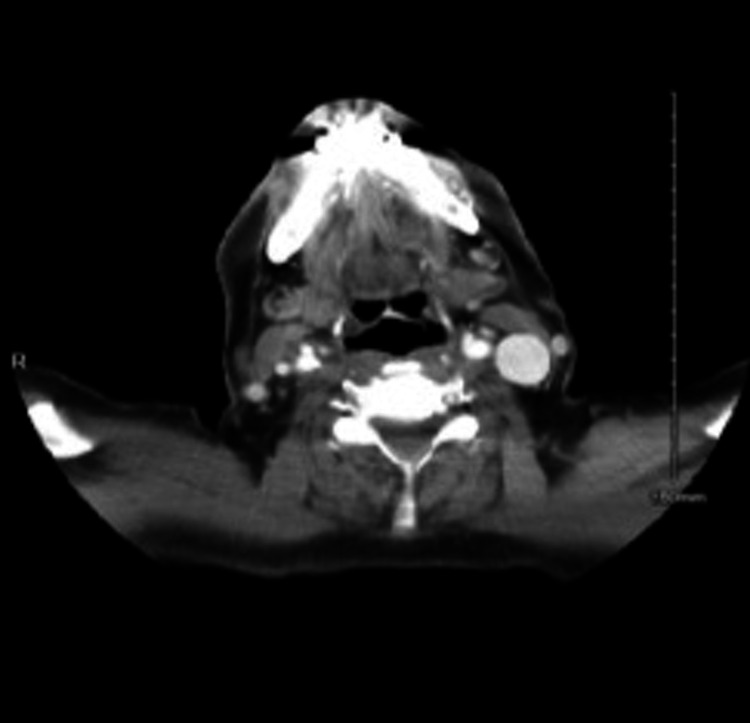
Post-Op CT scan. Clear hypopharynx with no sign of recurrence.
